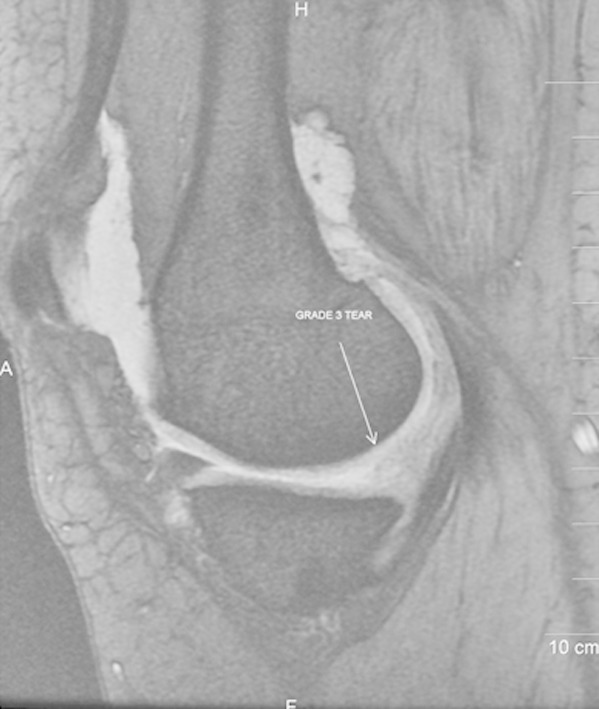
Sagittal T2-weighted MR image revealing complex meniscal tear in the posterior horn of medial meniscus seen extending to the articular surfaces. The tear was not recognized at arthroscopy performed 16 days after MR imaging and therefore constituting a false-positive diagnosis at MR.
